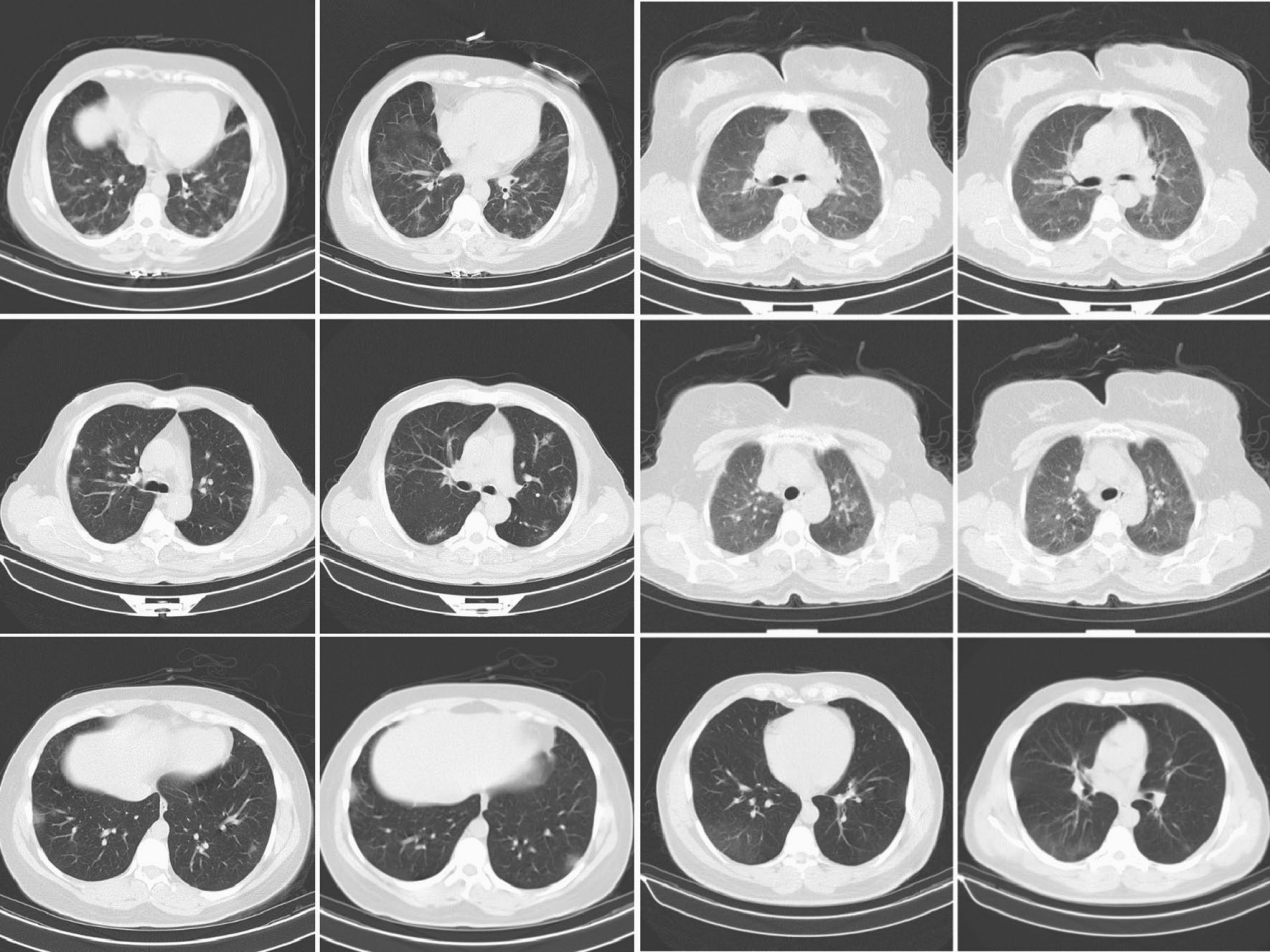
Sample lung CT scan images from dataset 4.
